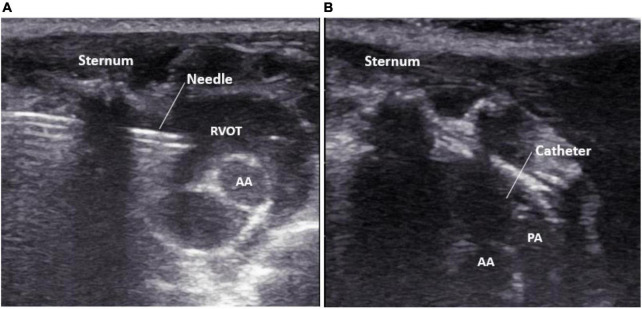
Real-time display of needle tip guided by high-frequency ultrasound. (A) The puncture needle tip is located in the right ventricular outflow tract; (B) the catheter tip is located in the pulmonary artery. PA, pulmonary artery; AA, aorta; RVOT, right ventricular outflow tract.
